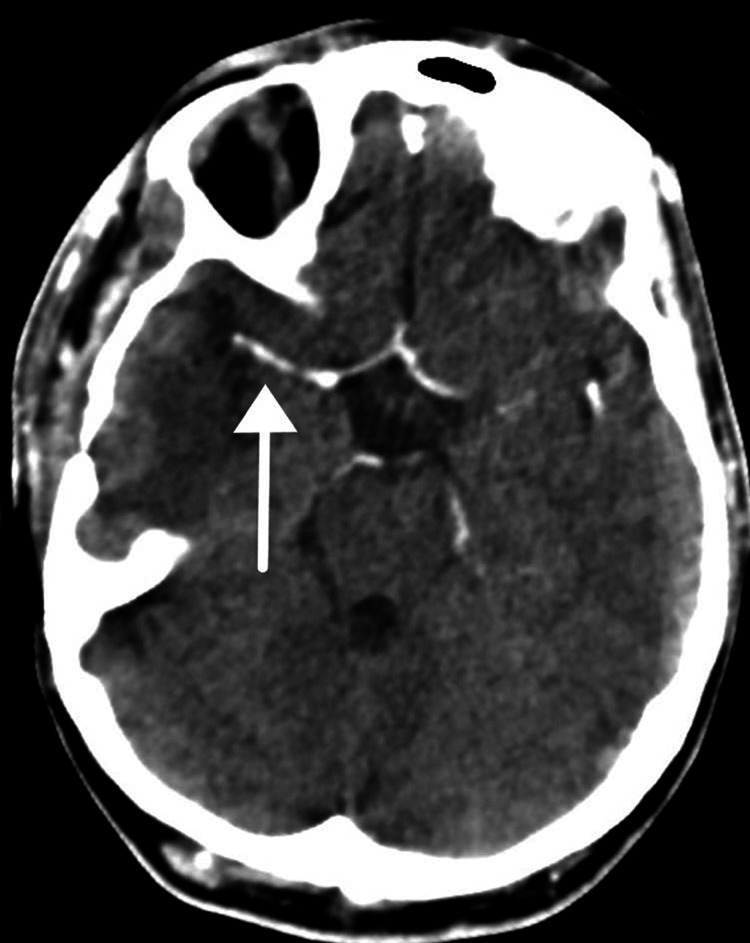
Computed tomography angiography of the brain Intracranial circulation shows irregularities and minimal to mild narrowing of the M1 segment of the right middle cerebral artery and the A1 segment of the right anterior cerebral artery.
